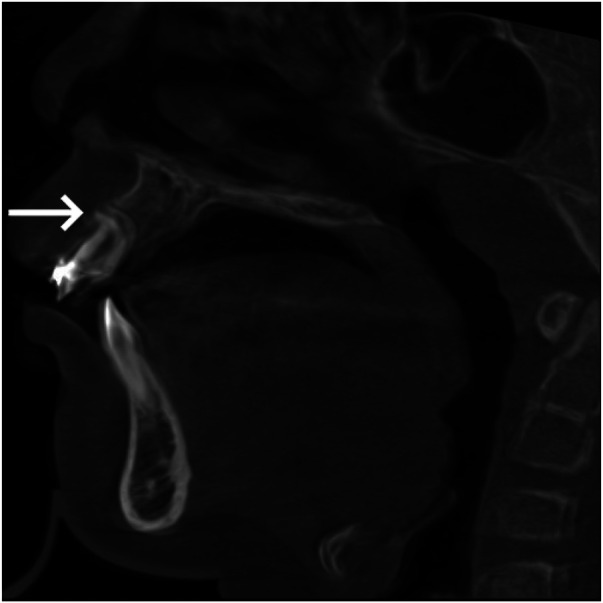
Post-treatment CBCT. Tooth 11 maintained a crown-root angulation of approximately 90 degrees, with radiographic evidence of fenestration in the apical region (white arrow).
